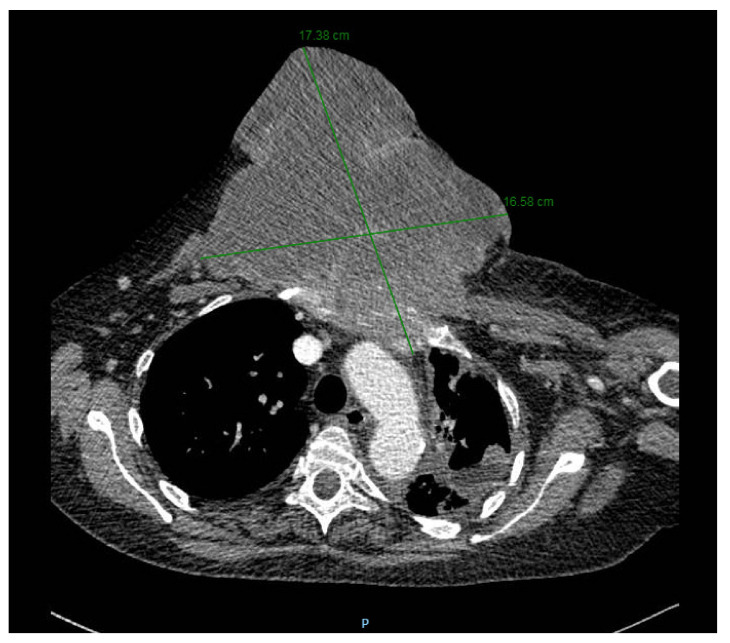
Preoperative CT scan (transversal view).
